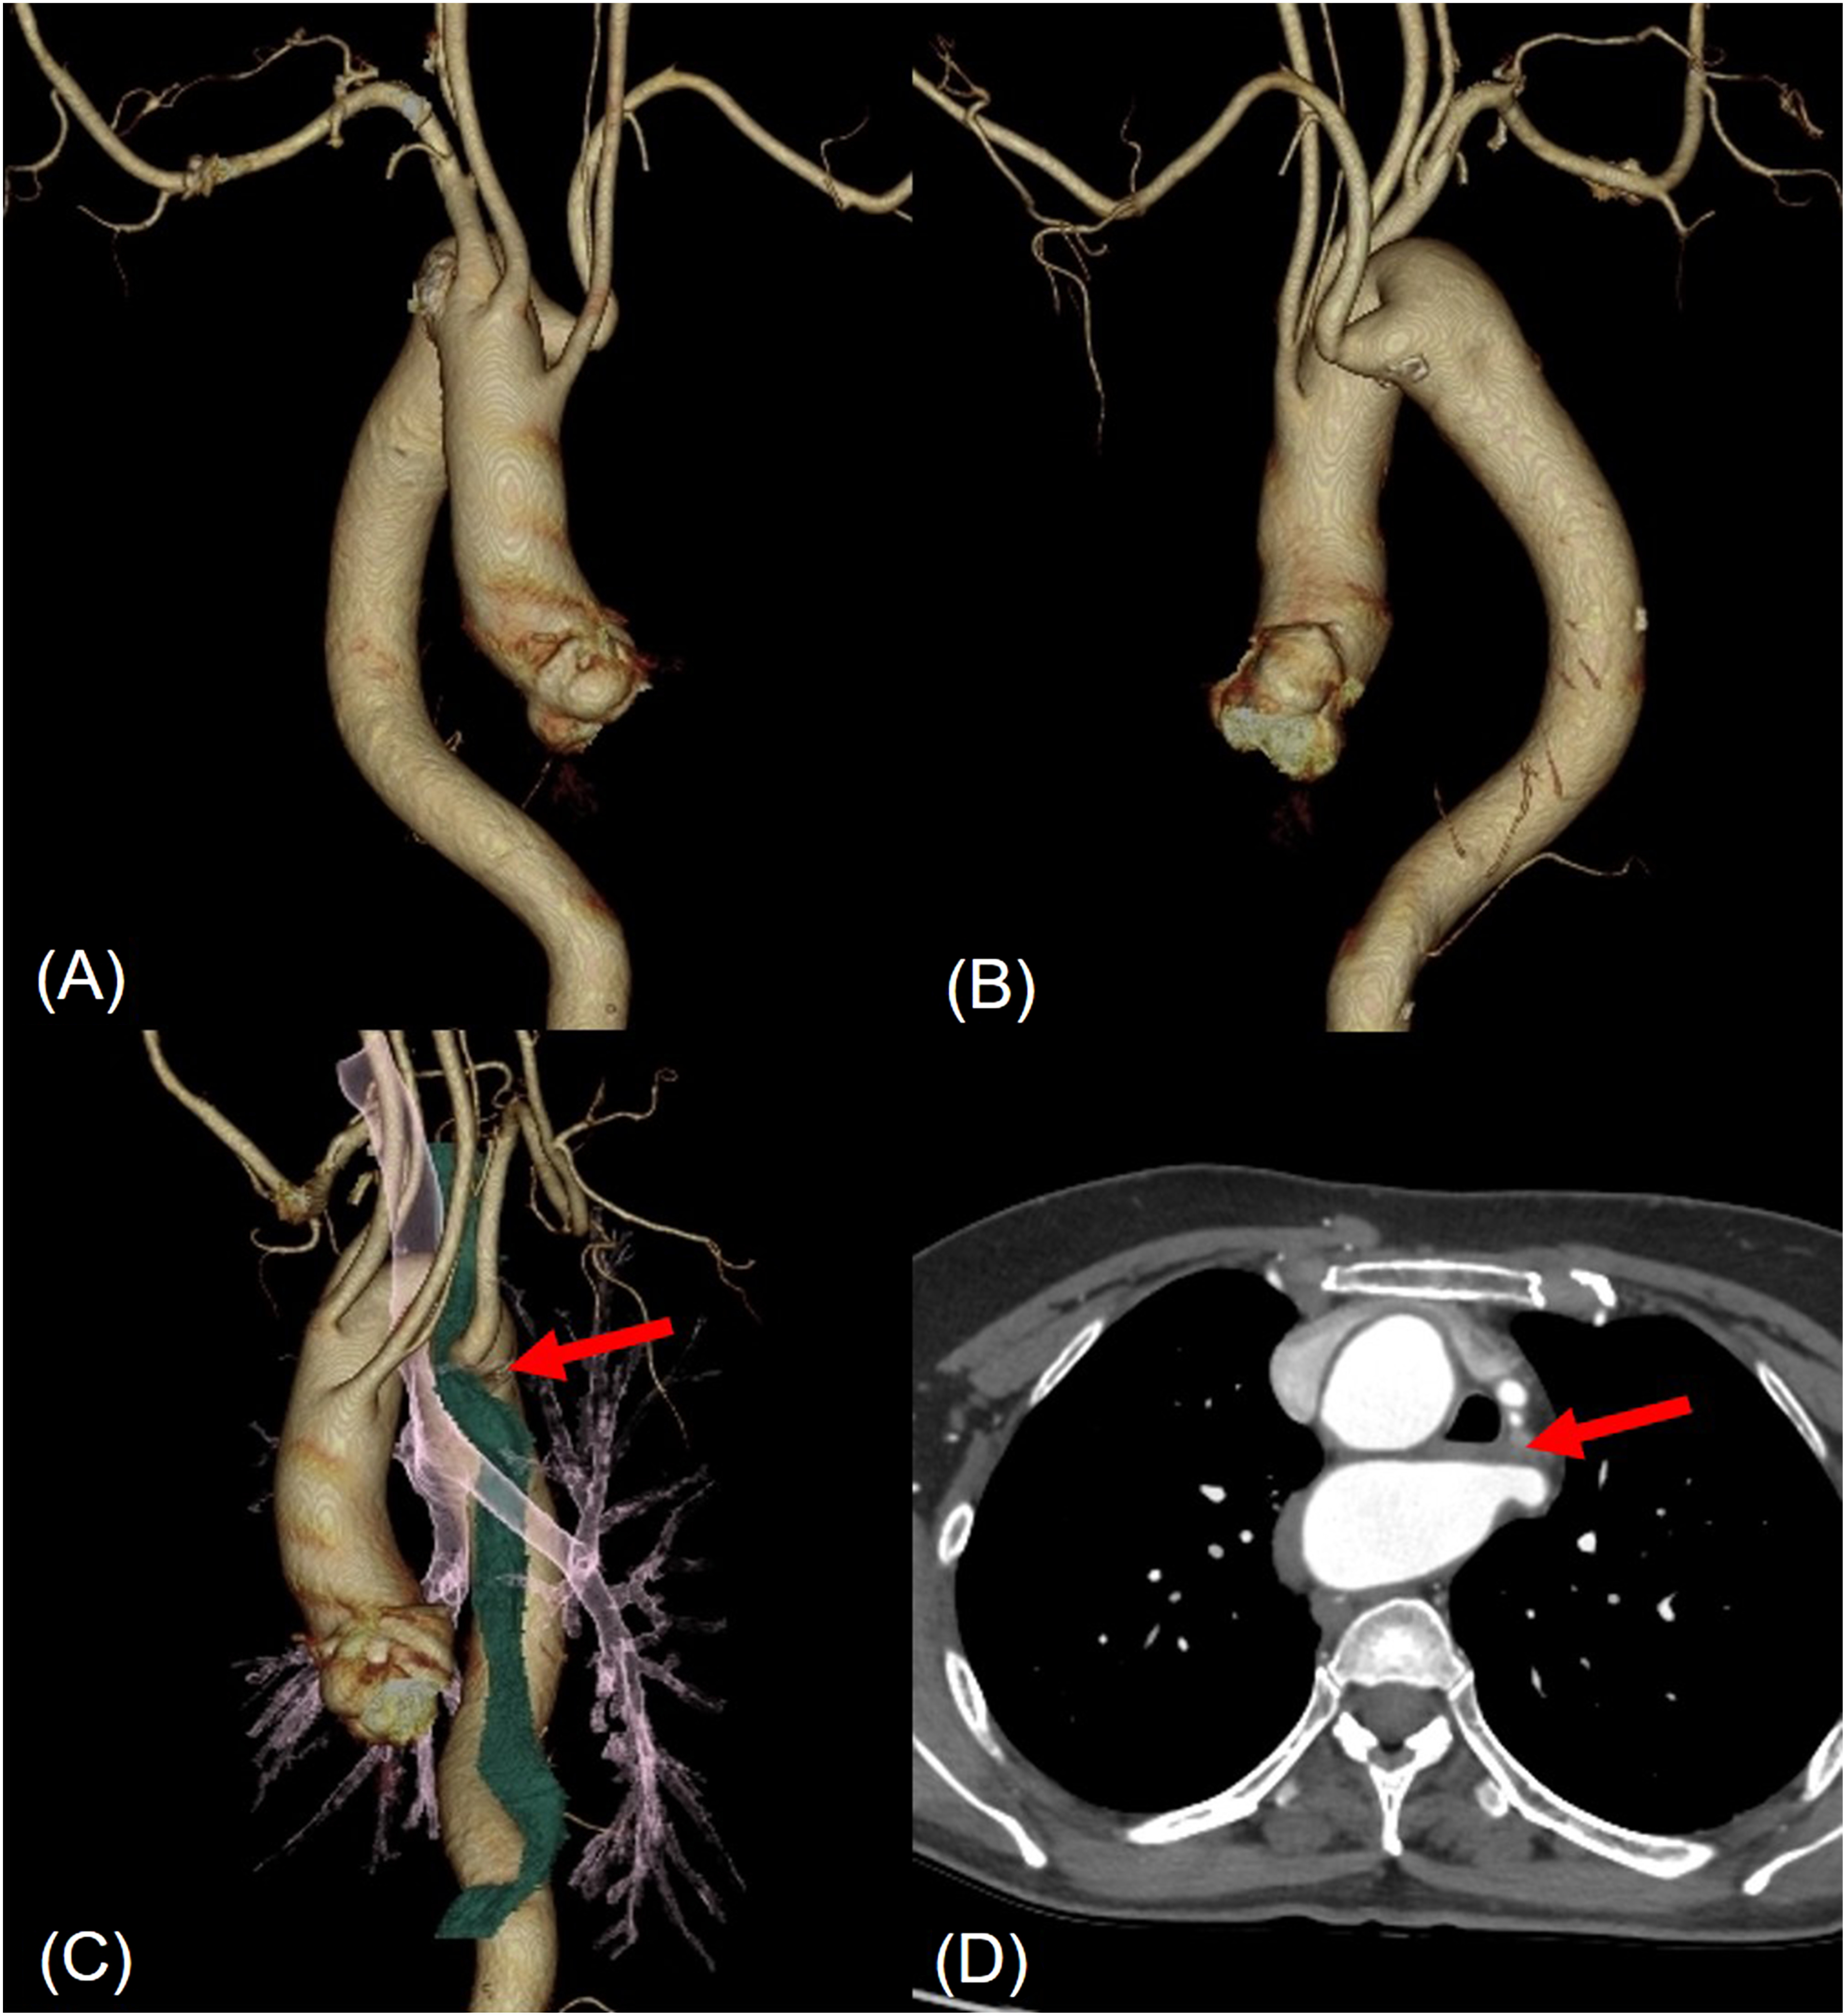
Fig. 1 Preoperative volume rendering computed tomography (CT) image of thoracic aorta. (A) Frontal view. (B) Left lateral posterior view. (C) Left lateral frontal view of the reconstructed three-dimensional CT showing the compressed esophagus (red arrow). (D) Transverse CT image of the aberrant left subclavian artery compressing the esophagus (red arrow).
